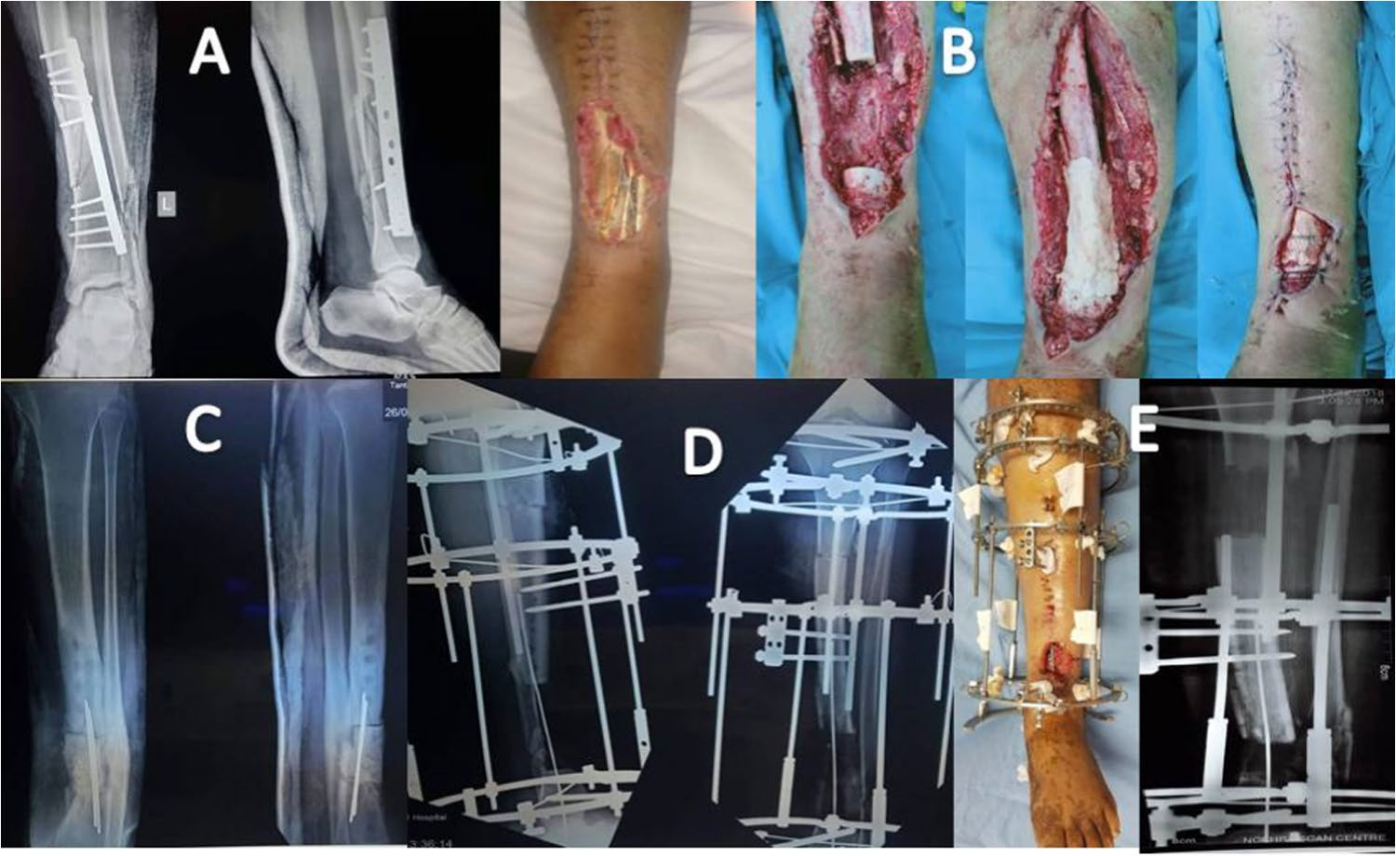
Case of infected nonunion of distal tibia over plate. A Ap and lateral X-ray of the Lt leg. Note the soft tissue defect. B Phase of debridement and management of the dead space. C Application of PMMA on double parallel K-wire. D, E Bone transport through the induced membrane chamber
